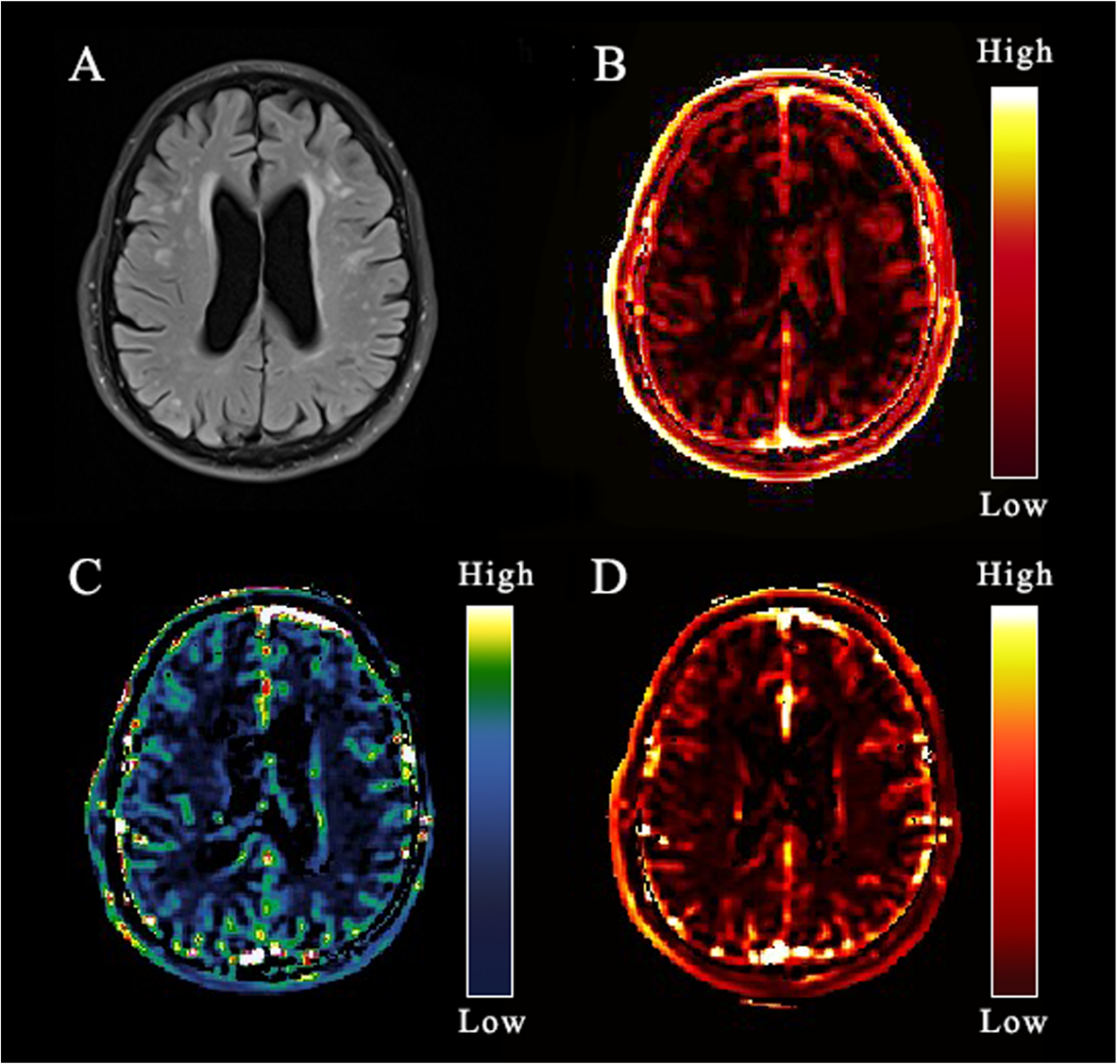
An example map. a Axial fluid-attenuated inversion recovery (FLAIR) image of a79-year-old man; b BBB leak (K_trans_) map; c Area under the leakage curve (AUC) map; d Fractional blood plasma volume (V_p_) map
